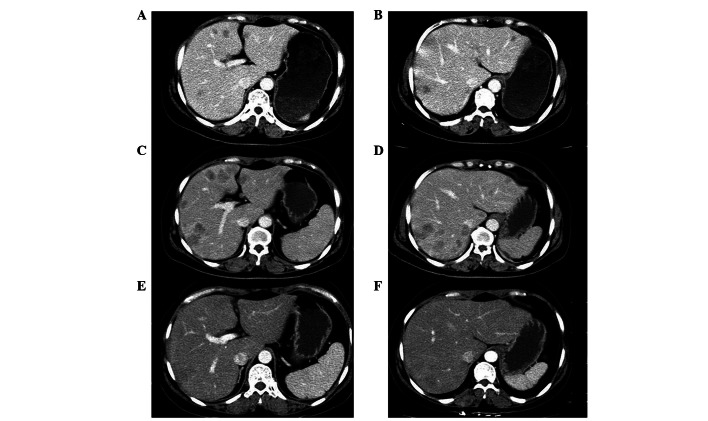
CT scans of liver metastases in a patient with a poorly differentiated serous papillary carcinoma of the right Fallopian tube. (A and B) At diagnosis of the metastases (March 2010); (C and D) after 2 cycles of second-line chemotherapy with topotecan (August 2010); (E and F) after 3 cycles of third-line treatment with gemcitabine plus cisplatin (October 2010). CT, computed tomography.
